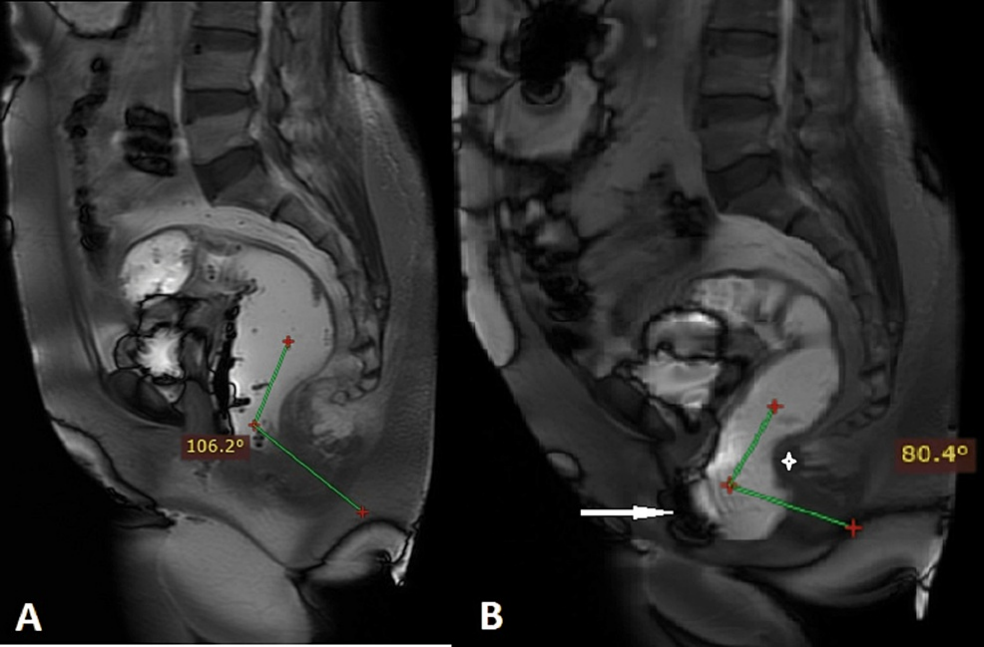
A 58-year-old lady presented with difficulty in defecation and suspected rectocele in clinical examination. Midline sagittal dynamic FIESTA MRI in rest (A) reveals a normal anorectal angle (106°). Midline sagittal dynamic FIESTA MRI in maximal strain (B) reveals a reduction in anorectal angle (80°) instead of an increase with prominent puborectalis sling impression on the posterior rectal wall (asterisk), indicating puborectalis dyssynergia. Anterior rectocele is also noted (white arrow). FIESTA: fast imaging employing steady-state acquisition.
